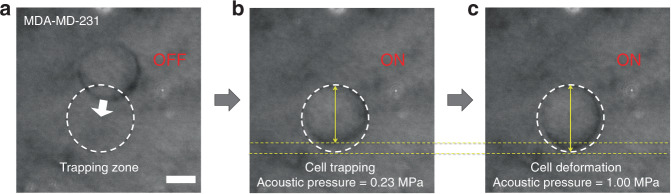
Cell trapping and deformation by the SBAT. a Ultrasound was off, and a MDA-MB-231 was located out of the trapping zone. b The cell was trapped at the trapping zone by the SBAT. c Cell membrane deformation under acoustic pressure. Scale bars indicate 10 μm
